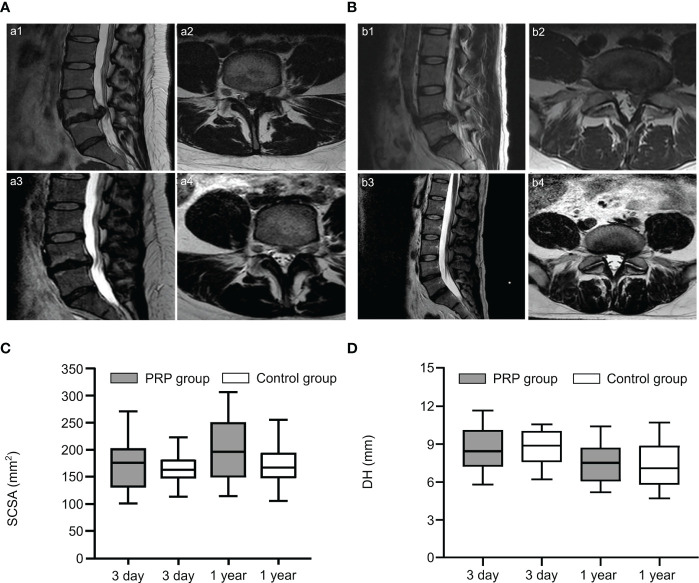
PRP treatment of lumbar disc herniation. (A, B) Sagittal and axial slices showed significant disc resorption before and after epidural injection of PRP in two patients. Sagittal (a1, b1) and axial (a2, b2) MRI images showed lumbar disc herniation in two patients before treatment; Sagittal (a3, b3) and axial (a4, b4) images showed significant disc resorption after treatment. (C, D) Comparison of spinal cord cross-sectional area (SCSA) and disc height (DH) on MRI at 3 and 1 years after treatment. Reproduced with permission from (60, 61).
